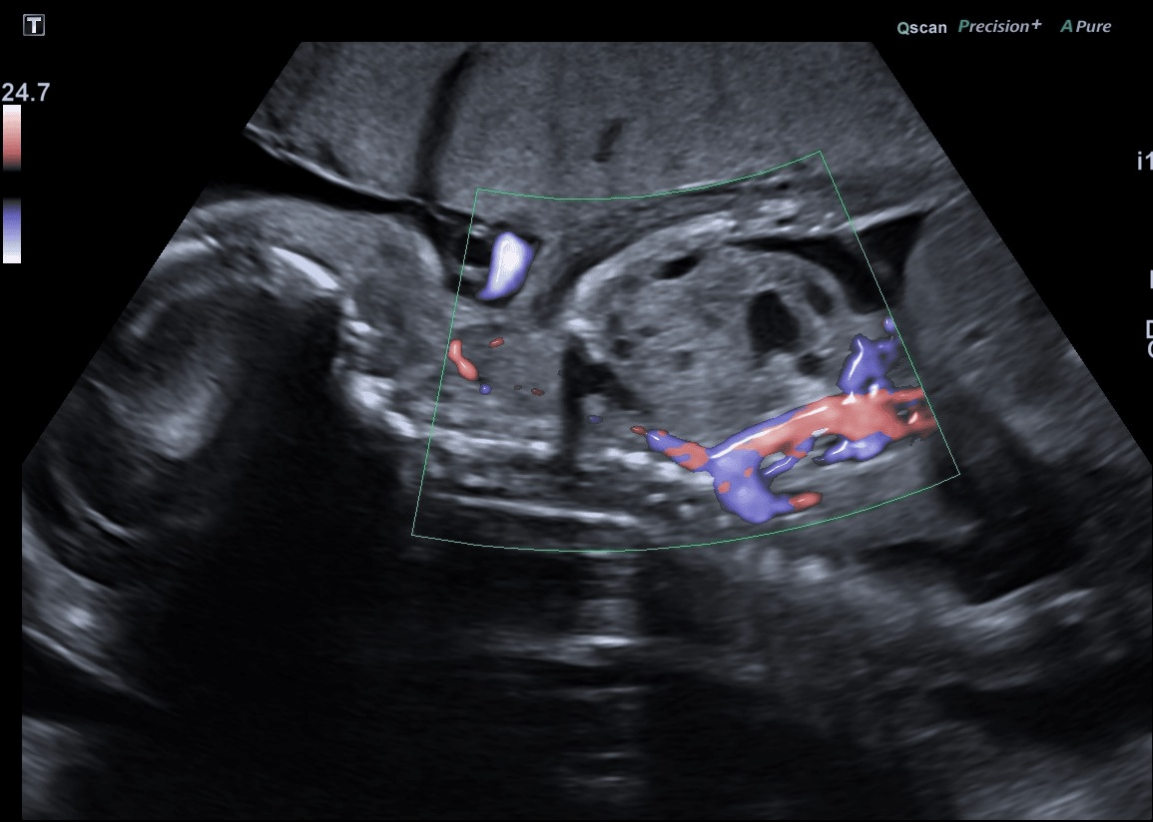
Sagittal ultrasound view of the fetal thorax and abdomen demonstrating the mediastinal mass with no color Doppler flow.
